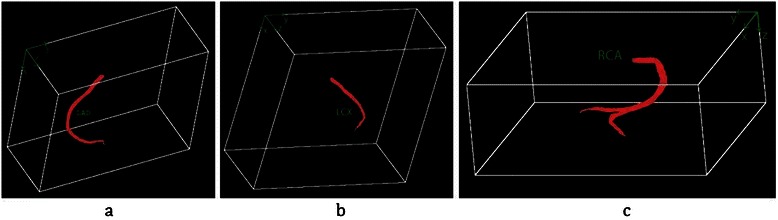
The result of coronary artery labeling and 3D reconstruction in CTA. Typical examples of LAD, LCX and RCA reconstructions are shown in (a), (b), and (c), respectively.
